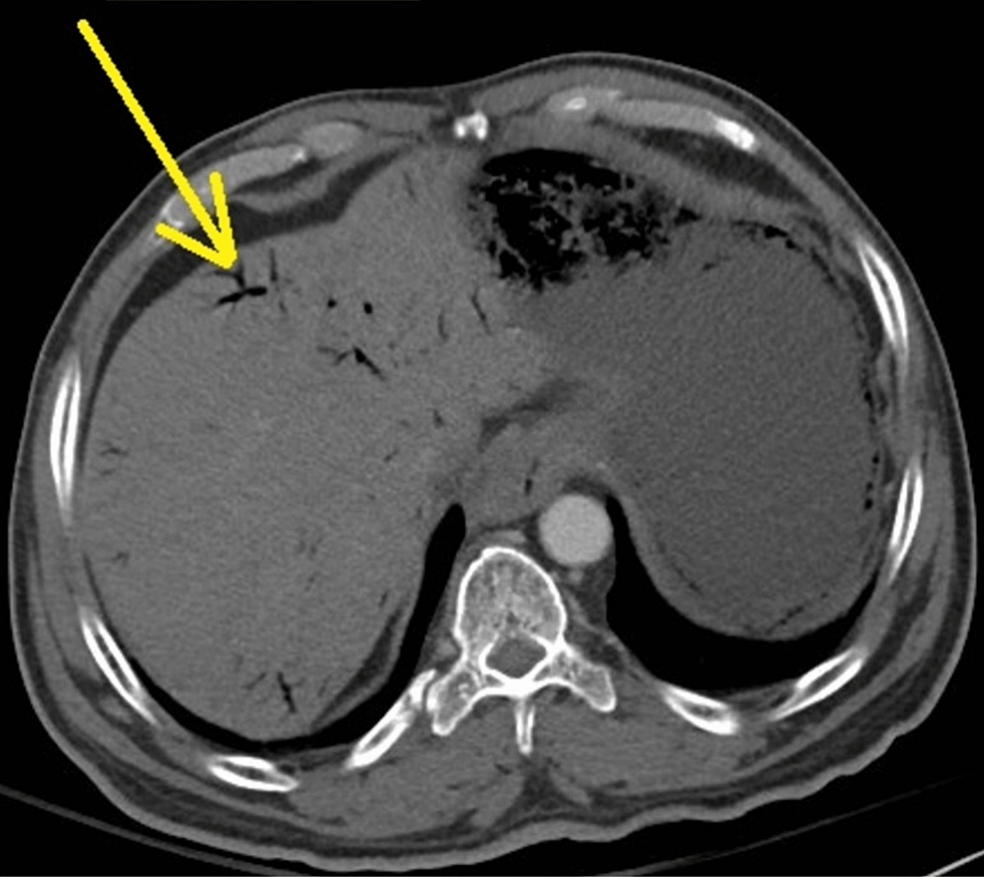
Abdominal CT scan showing portal vein pneumatosis (yellow arrow)
